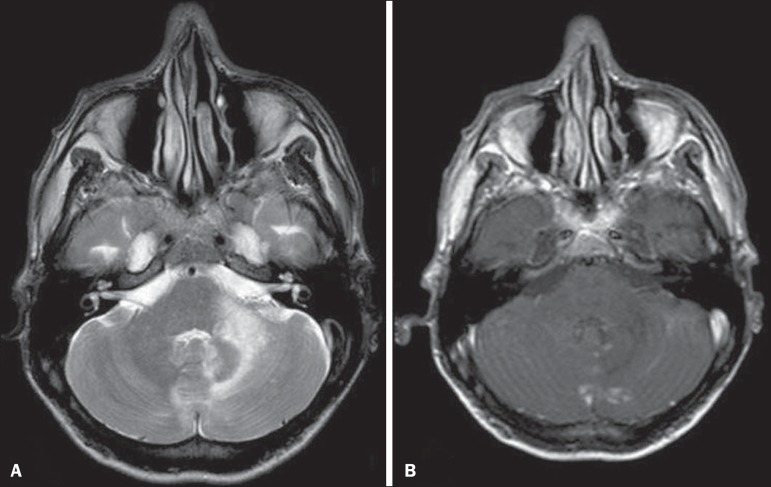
Progressive multifocal leukoencephalopathy. Axial T2-weighted MRI
